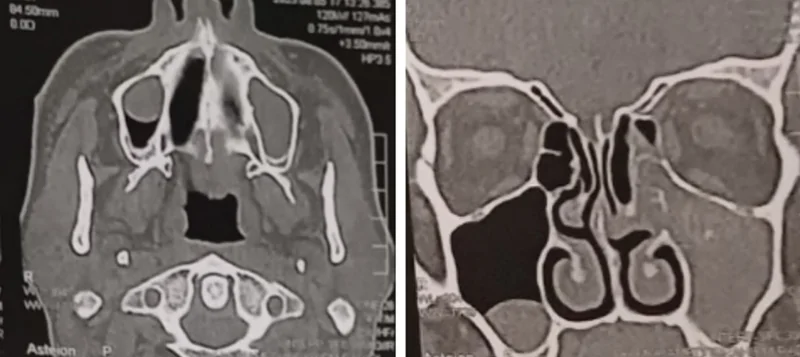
Axial and coronal CT nose and PNS showing left ACP, right antral cyst and deviated nasal septum to right side.
